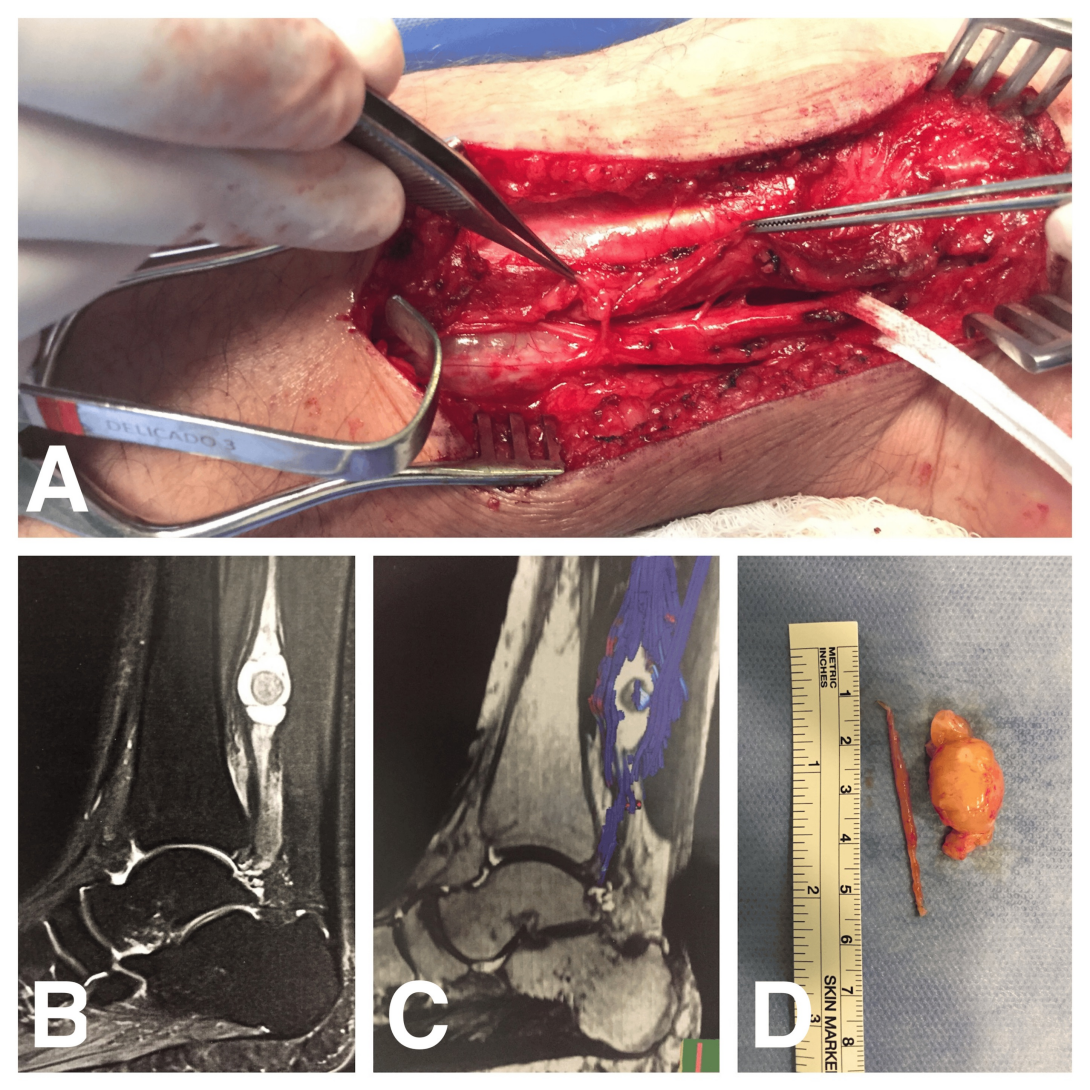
Case 1: Intraoperative views showing complete tarsal tunnel decompression and tumor resection (A, D); sagittal T2-weighted MRI (B) and MR neurography (C) images demonstrate a distal leg tibial nerve schwannoma displacing nerve fascicles laterally MRI, magnetic resonance imaging; MR, magnetic resonance
